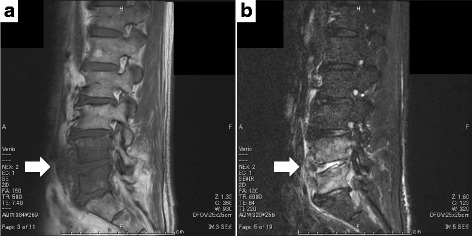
Magnetic resonance imaging of the patient’s spine. Magnetic resonance imaging shows low intensity at the L4 and L5 vertebral bodies and L4–L5 disc space in T1-weighted images (a) and hyperintensity in T2-weighted images (b) 2 days before admission (*white arrows*)
